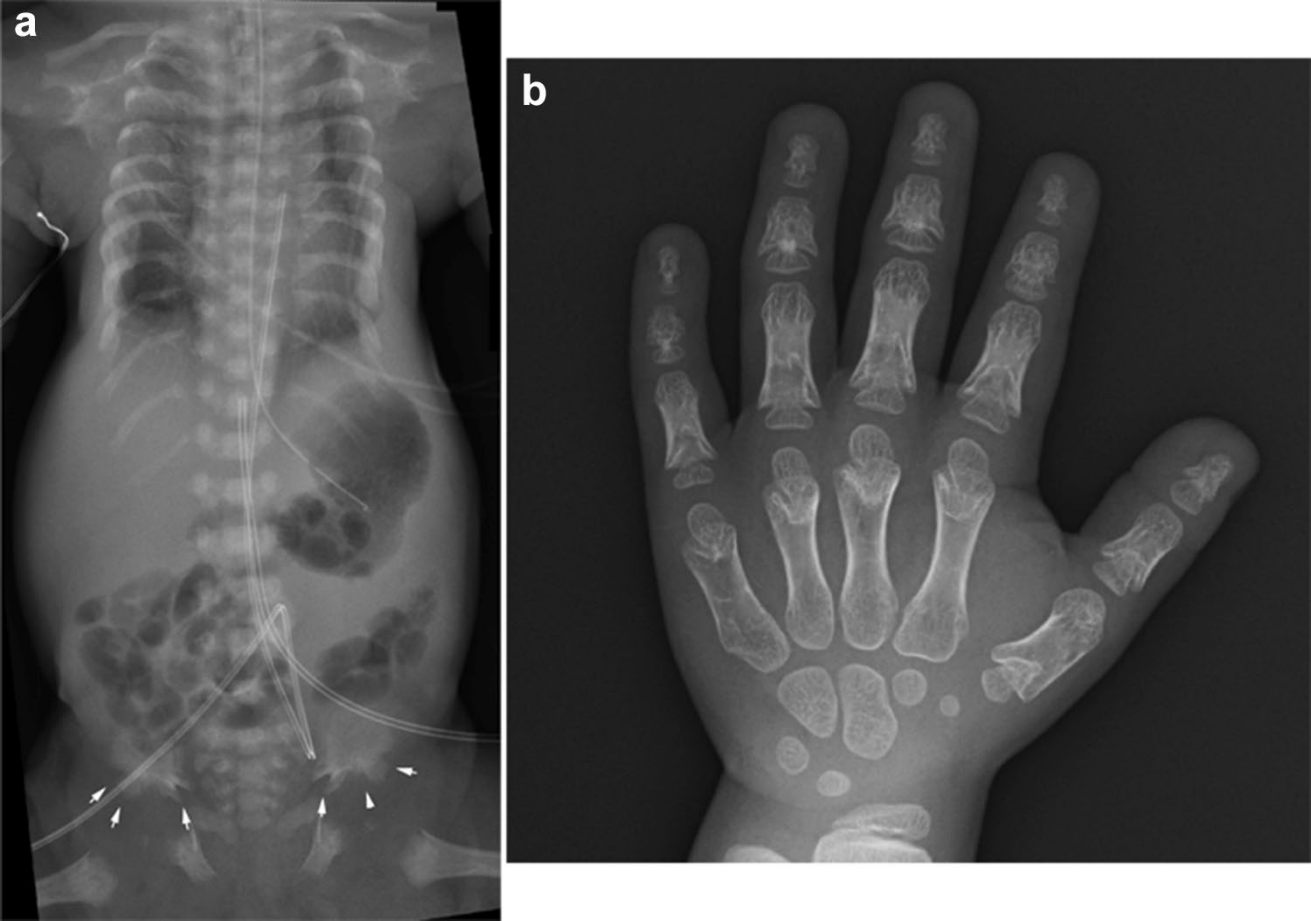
a A neonate with Jeune asphyxiating thoracic dysplasia. Radiograph shows markedly short ribs, and trident-shaped ilia (arrows). Severe brachydactyly without polydactyly were also noted (not shown). Premature ossification of the proximal femoral epiphyses is distinctive. As opposed to achondroplasia, there is no caudally decreased interpedicle distance or ovoid lucency of the proximal femora. (Reprinted from Handa, A, et al. Jpn J Radiol. 2020;38(3):193–206). b A child with the same diagnosis. Radiograph shows cone-shaped epiphyses involving both the phalanges and the metacarpals. Cone-shaped phalangeal epiphyses and inverted tear-shaped metacarpal epiphyses invaginate into cupped metaphyses. Premature fusion of the growth plate is also seen in the middle and distal phalanges. (Reprinted from Handa, A, et al. Jpn J Radiol. 2020;38(3):193–206)
